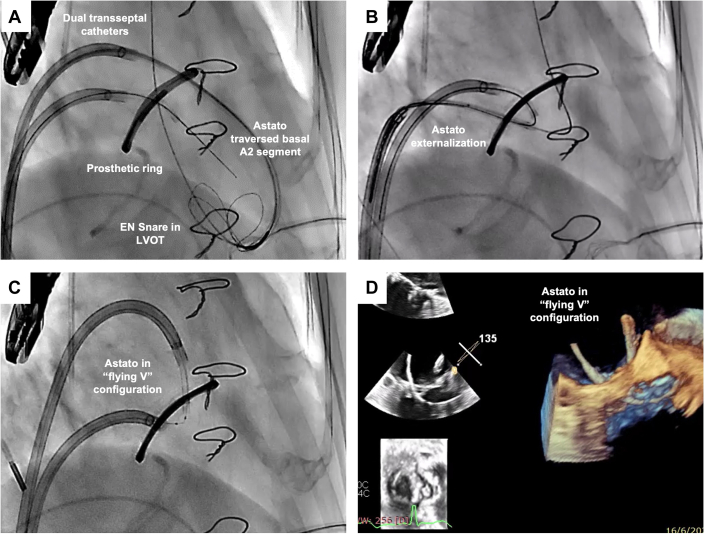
Antegrade Base-to-Tip LAMPOON Technique (A) Two transseptal steerable sheaths are placed in the left atrium, one for delivering Astato wire for traversing the A2 segment of the anterior mitral valve leaflet; and another one for positioning the EN Snare in the LVOT. (B) After leaflet traversal, the Astato is snared and externalized. (C) Astato wire in “flying V” configuration is used to lacerate the anterior mitral valve leaflet. (D) “Flying V” shown on transesophageal echocardiography. Abbreviations as in Figure 1.
